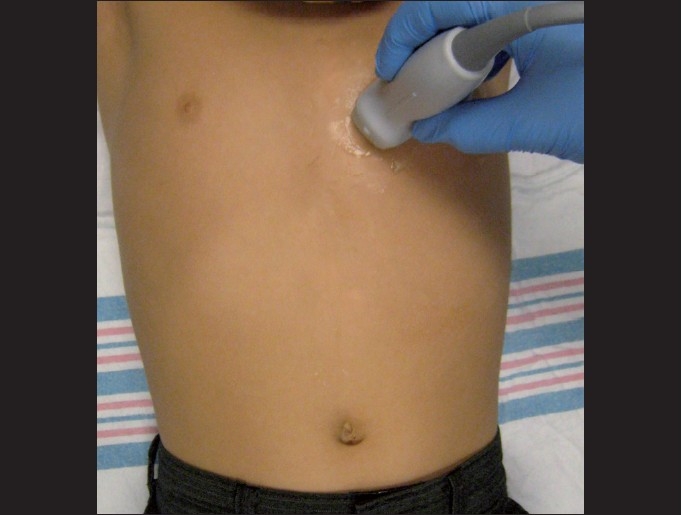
The parasternal short-axis view. The transducer is oriented with the probe marker toward the patient’s right hip, or in the 7:00 direction. This is a 90-degree rotation from the parasternal long-axis view
